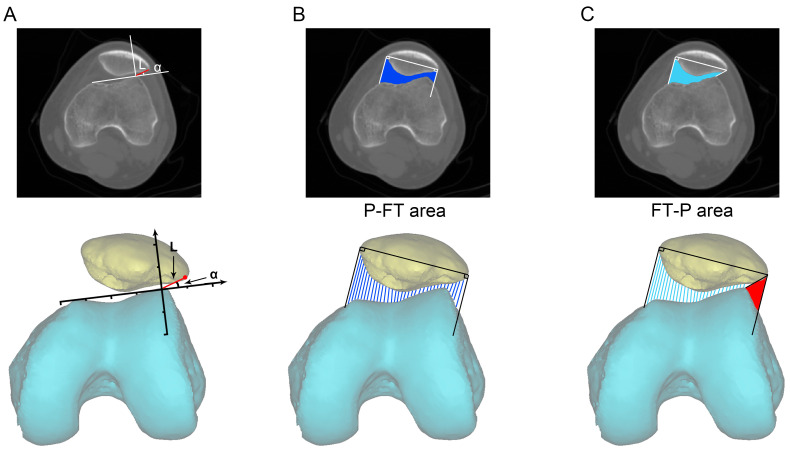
Two newly designed assessment indicators for the patellofemoral joint. (A) In an axial CT image of the knee joint, on the plane connecting each of the highest points of the medial and lateral femoral condyles, a connecting line was created between the outermost point of the patella and the highest point of the lateral femoral condyle. The length of the line was considered as L, and the angle between this line and the line connecting each of the highest points of the medial and lateral femoral condyles was regarded as α (with the line connecting each of the highest points of the medial and lateral femoral condyles as the *X*-axis and the line from the highest point of the lateral condyle as the *Y*-axis; this allowed us to establish a coordinate system for L and α). (B) Patella-femoral trochlear (P-FT) area: in an axial CT image of the knee joint, the longest axis between the medial and lateral sides of the patella was first drawn on the plane connecting each of the highest points of the medial and lateral femoral condyles, and two lines perpendicular to the long axis were created at the outermost and medial points of the patella. The cross-sectional area from the lower edge of the patella to the femoral trochlear groove between two vertical lines was measured (the area marked in blue). (C) Femoral trochlear-patella (FT-P) area: in the axial CT image of the knee joint, the longest axis between the medial and lateral sides of the patella was first drawn on the plane connecting each of the highest points of the medial and lateral femoral condyles. A line perpendicular to the long axis was then drawn at the medial point, and a connecting line was created between the outermost point of the patella and the highest point of the lateral femoral condyle. The cross-sectional area from the lower edge of the patella to the femoral trochlear groove between the two connecting lines was then measured (the area shown in light blue). The area of the red region indicates the difference between the P-FT area and the FT-P area.
